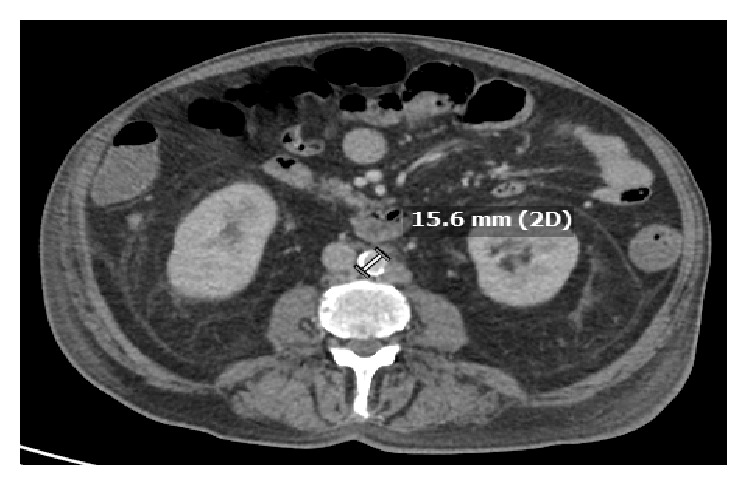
CT abdomen/pelvis with IV contrast showing the abdominal aorta measuring 15.6 mm at narrowest point.
